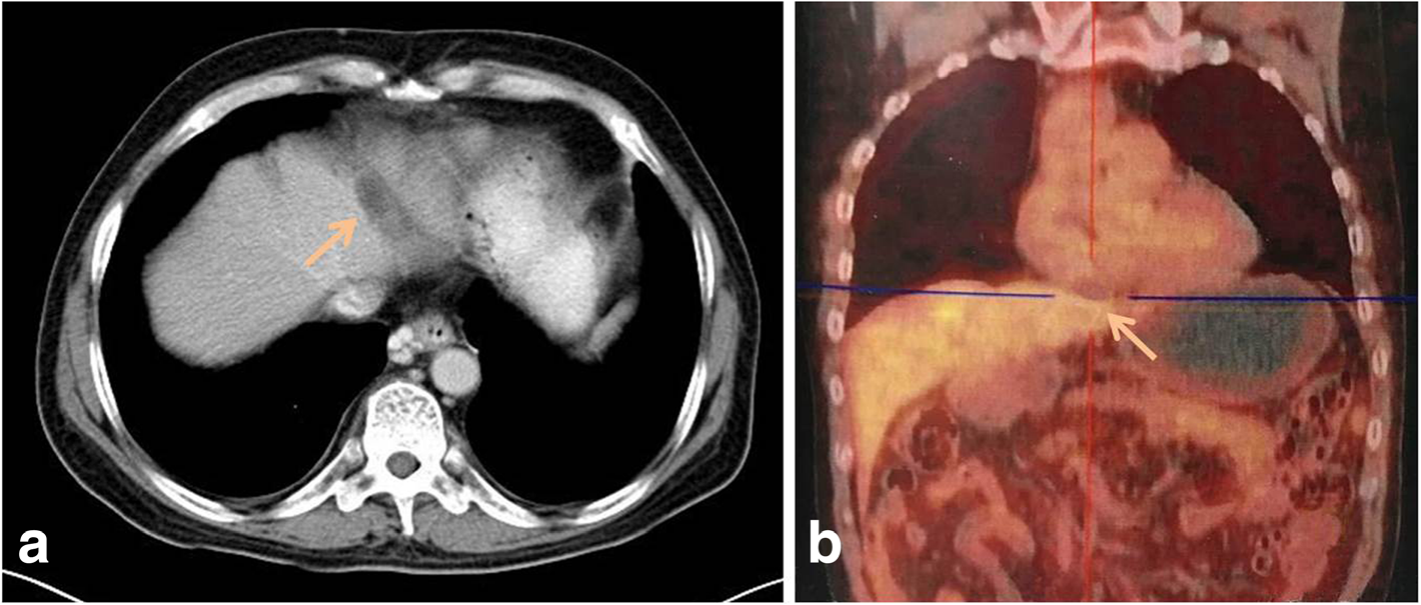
Follow-up imaging examination after cryoablation. a Follow-up computed tomography scan 1 month later showed a 3.5 × 1.8 × 1.7 cm ablated zone, with no evidence of recurrence. b Follow-up positron emission tomography–computed tomography examination six month later showed no evidence of recurrence. The *arrows* indicate the ablated zone
